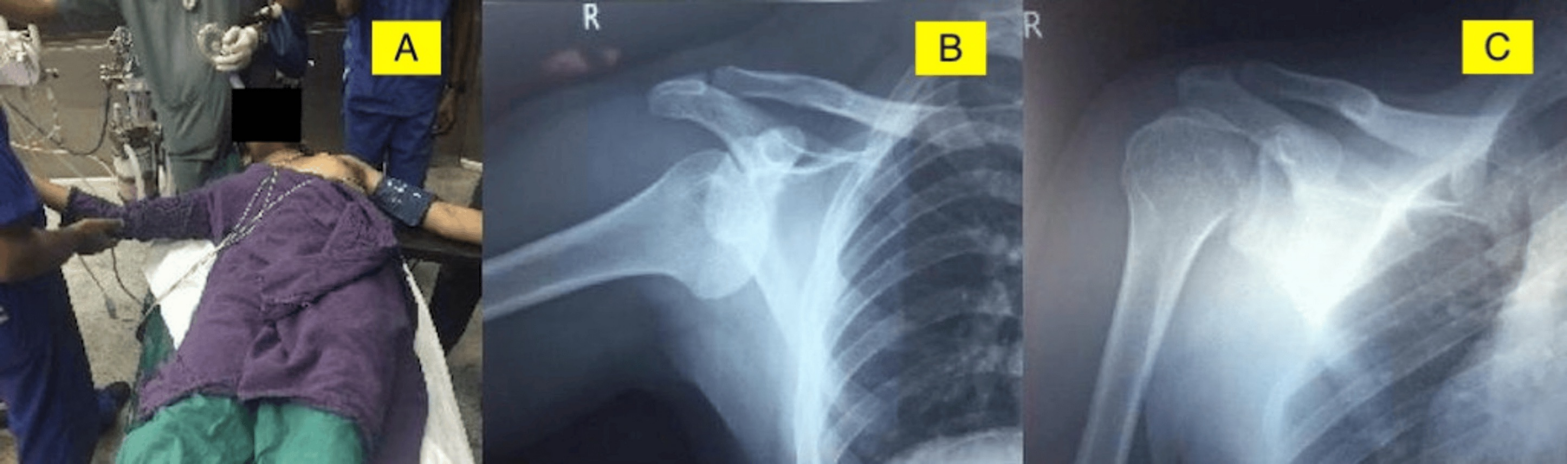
Case 1 A) The right arm and shoulder are in an abducted position with inferior shoulder dislocation. B) Radiograph showing right sub-coracoid inferior shoulder dislocation. C) Post-reduction radiograph showing shoulder in situ after 'two-step manoeuvre'.
